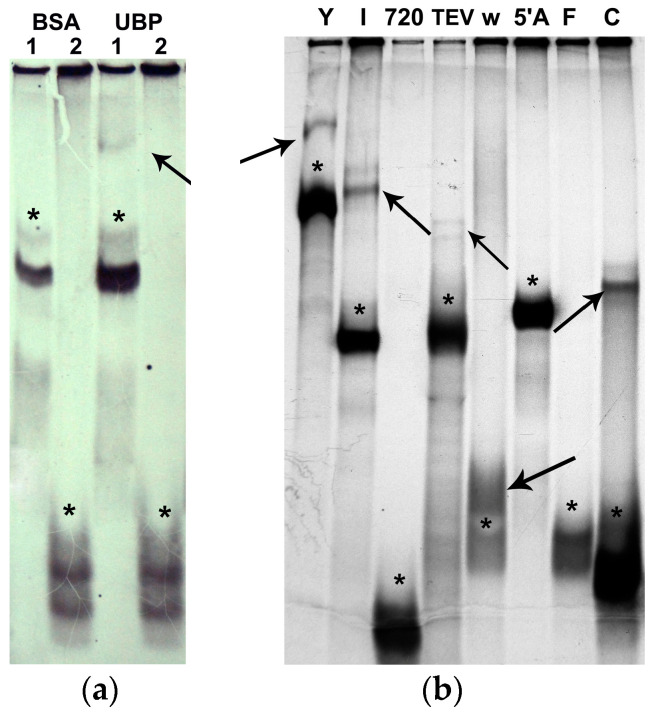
Results of gel-shifting assay in 10% PAGE on X-ray film. (a) Gel shifting of 5′PVM (lane 1) and 5′εII (lane 2) with addition of BSA or AtUBP1b proteins; (b) gel shifting of Y (5′PVY), I (PVY1-132), F (PVY1-65), C (PVY66-132), 720 (PVY133-182), TEV (5′TEV), w (Ω-element), 5′A (5′CspA) with addition of AtUBP1b. Arrows show RNA–protein complexes; asterisk—RNA that was not bound.
